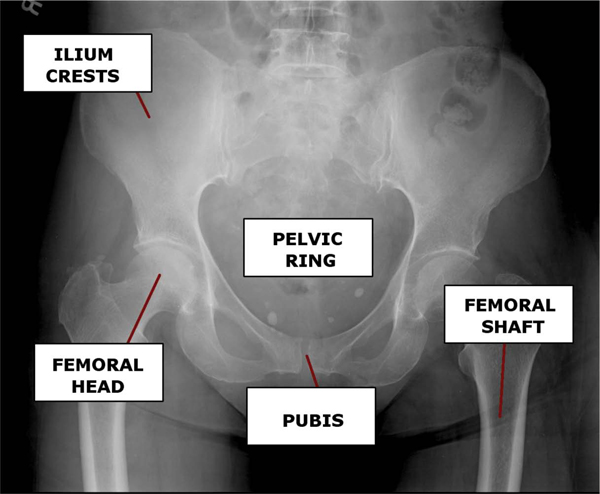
Bones of the Pelvis. An x-ray showing key structures of interest located within the human pelvis.
